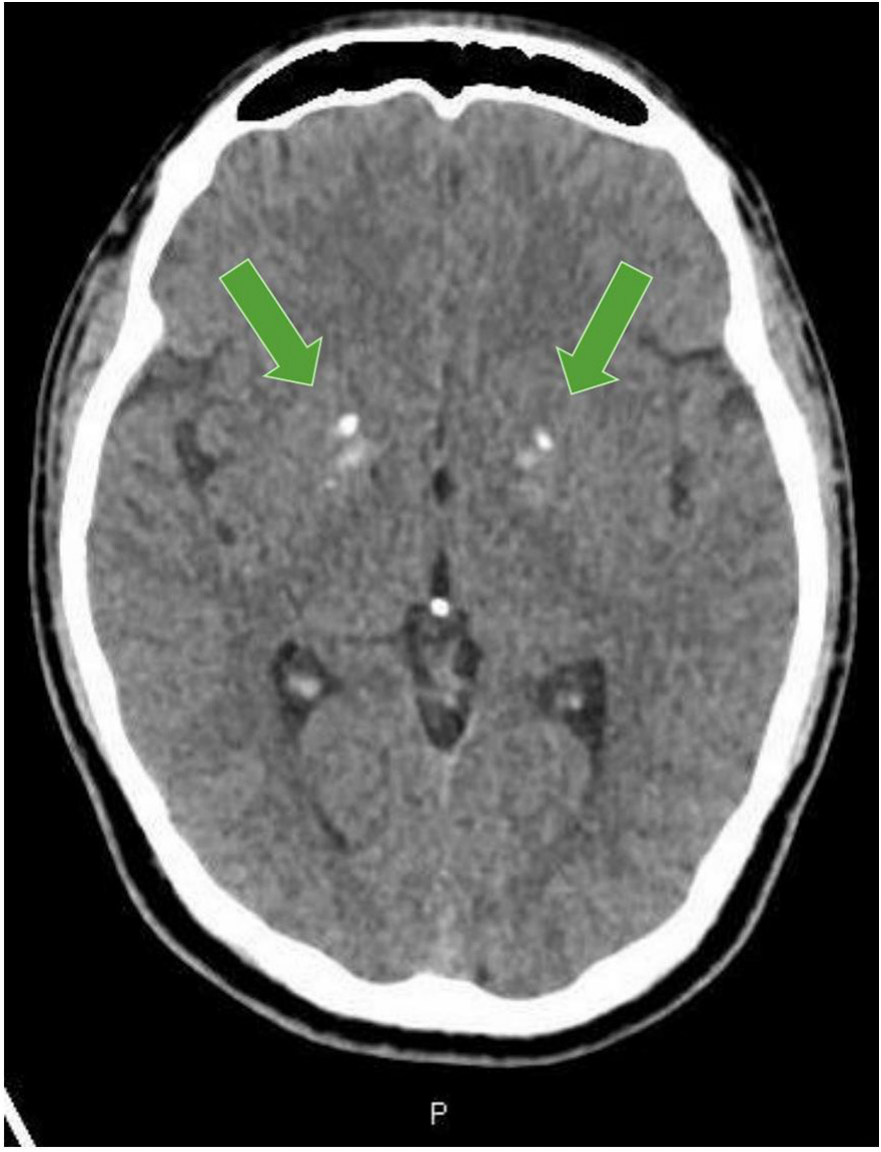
Head CT showing bilateral basal ganglia calcifications.
